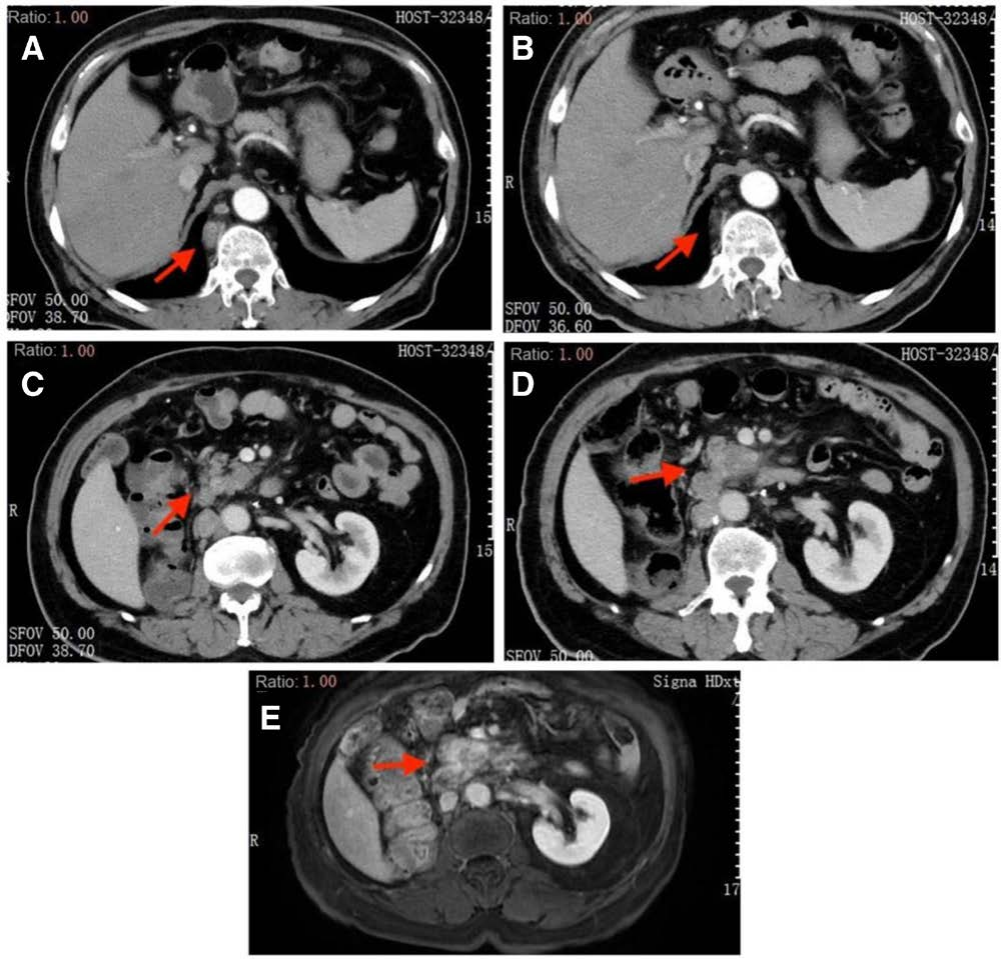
CT scanning images of the patient. The red arrow showed the major lesion. (A) CT scanning 7 months after radical therapy of renal carcinoma on December 15, 2020 showed enlargement of lymph nodes posterior to the right diaphragm angle. (B) CT scanning after 4 cycles of combination therapy by immunotherapy and targeted-therapy on March 30, 2021, showed substantial shrinking of the lymph nodes posterior to the right diaphragm angle. (C) Abdominal CT scanning on December 15, 2020, showed no abnormality in the pancreas. (D) CT scanning after 4 cycles of combination therapy by immunotherapy and targeted-therapy on March 30, 2021, showed substantial shrinking of lymph nodes posterior to the right diaphragm angle, but a low-density lesion appeared at the uncinate process of the pancreas. (E) Abdominal MRI examination showed the malignancy at the uncinate process of the pancreas. CT = computed tomography.
